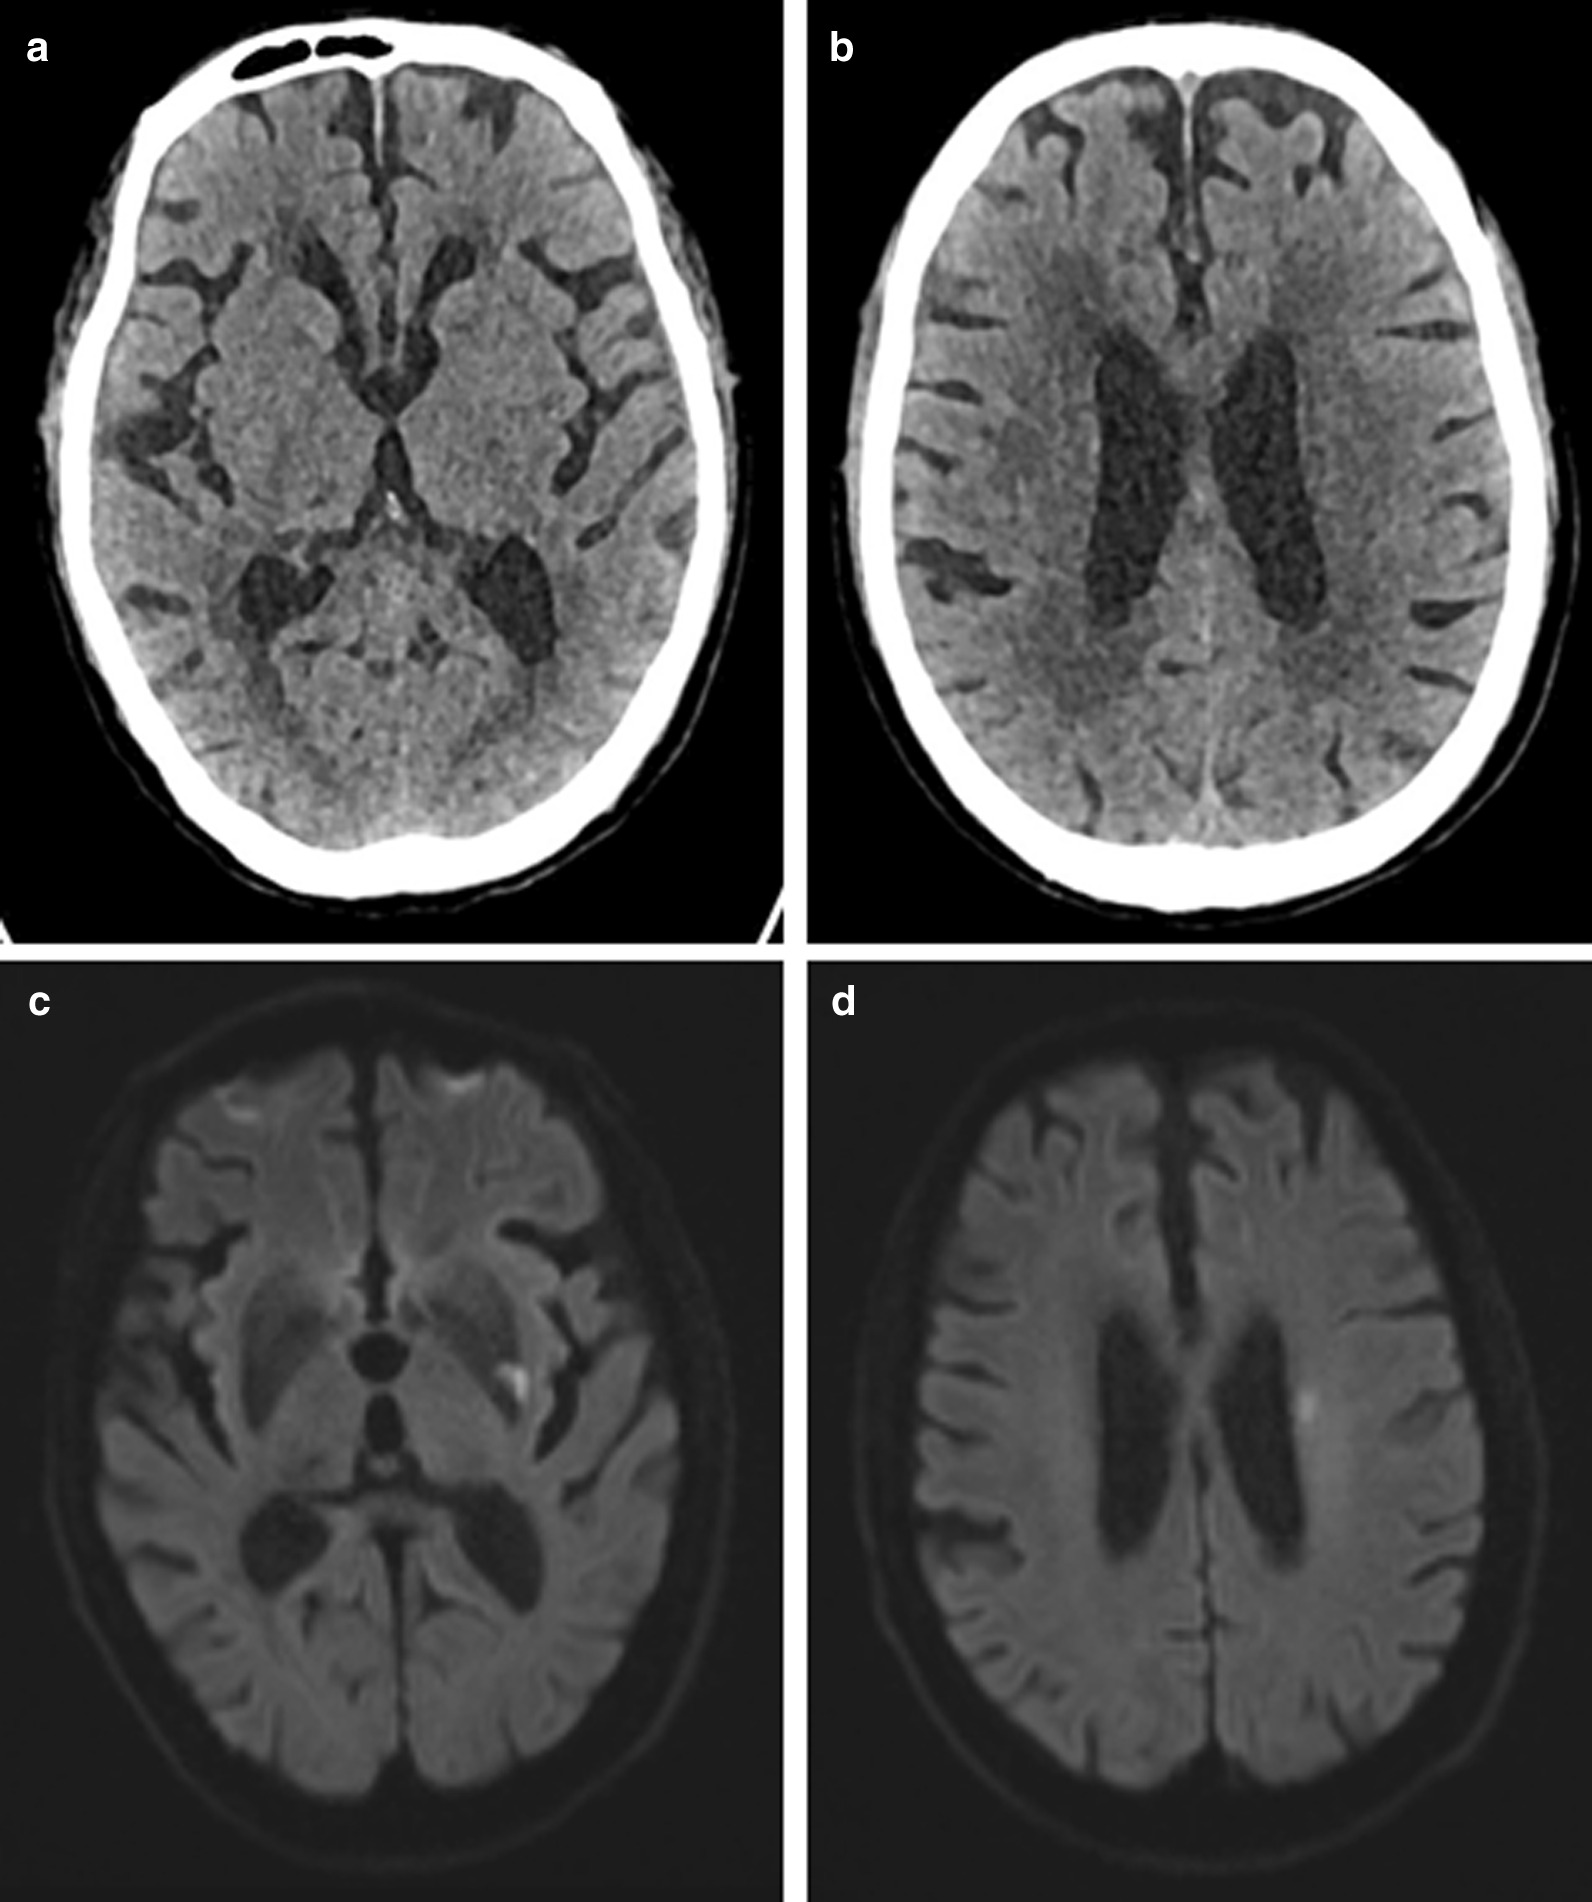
Patients with good clinical prognosis: a patient was admitted to the hospital 30 min after the onset of stroke. At admission, the patient's ASPECTS was 9 points, and the HU difference was 12.94. The infarct volume calculated on the DWI image at 7 days after thrombolysis was 4.14, and the 3-month mRS score was 2 points. a, b NCCT image on admission. c, d DWI image at 7 days after thrombolysis
